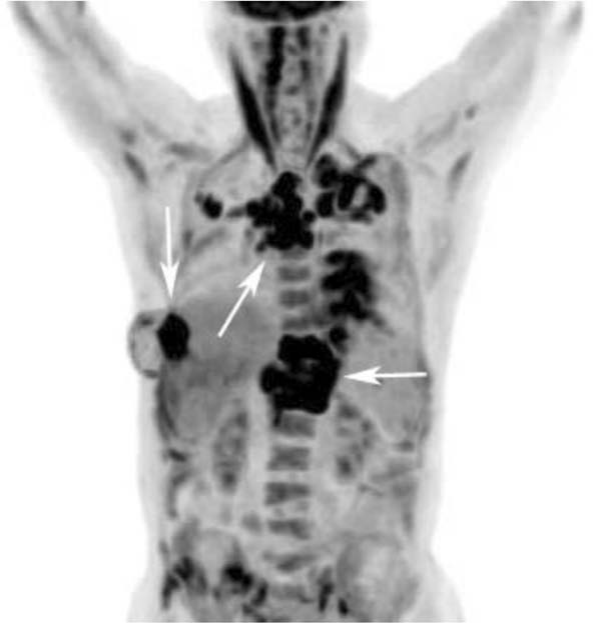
CT image of the patient with multifocal musculoskeletal tuberculosis. F-18 FDG-PET/CT images showed irregular increased FDG uptake (*arrowheads*) with maximum SUVs ranging from 10.2 to 18.5 and involving bilateral collarbones, ribs, thoracolumbar vertebrae, ilium, sacrum, pubis, hip and the left side of the femoral neck
